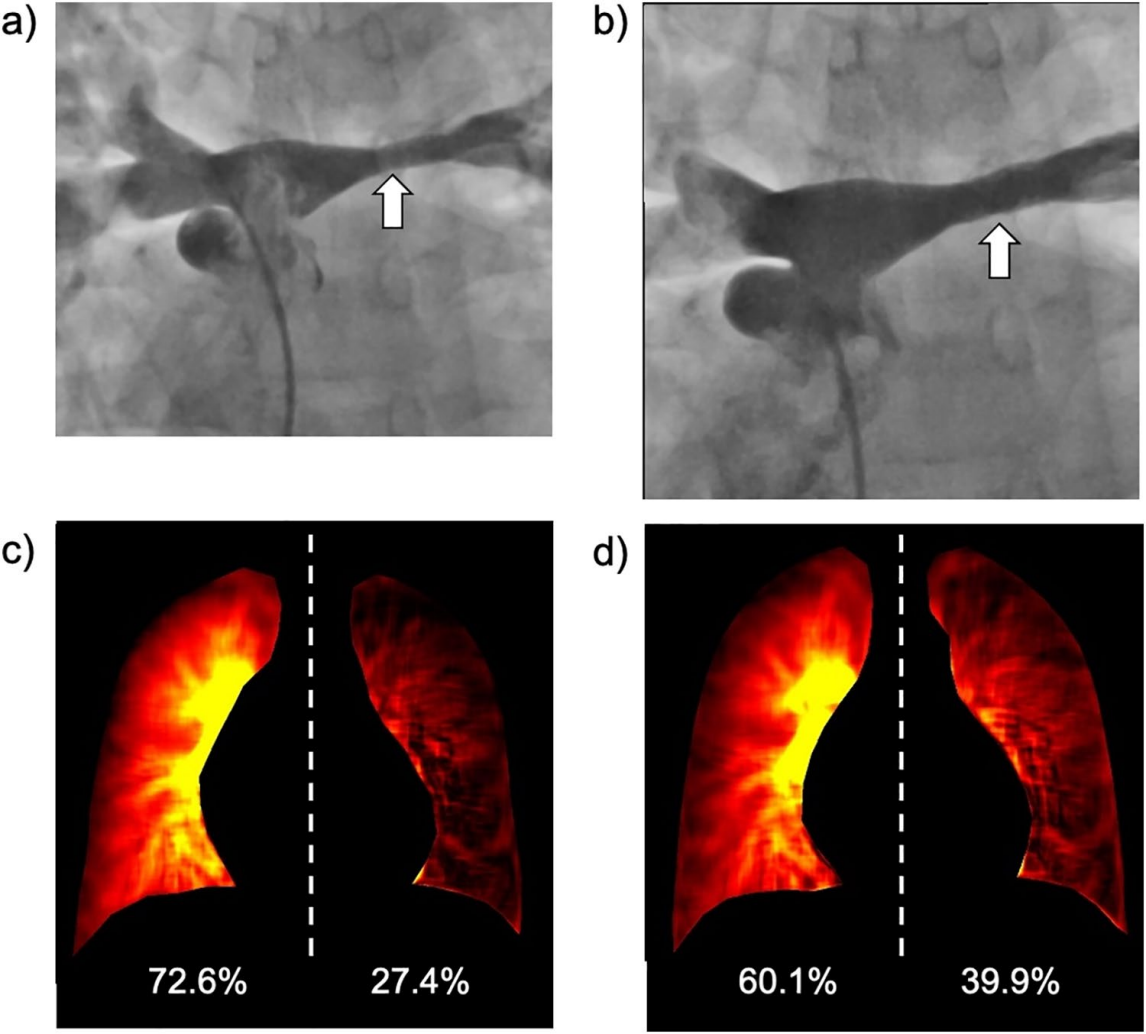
Images of a 17-year-old boy with repaired transposition of the great arteries before and after stent placement for the left pulmonary artery stenosis. The pressure gradient between the main and left pulmonary arteries was 13 mmHg before and 8 mmHg after treatment. Pulmonary angiography showing left pulmonary artery stenosis (a, arrow) and improvement after stent placement (b, arrow). Semi-quantitative evaluation of pulmonary perfusion using the perfusion map of dynamic chest radiography (c) showed similar values (right 72.6%, left 27.4%) to those of phase-contrast magnetic resonance imaging (76.0%, 24.0%) and perfusion scintigraphy (70.4%, 29.6%). The improvement in maldistribution after treatment is also demonstrated (60.1%, 39.9%) (d)
